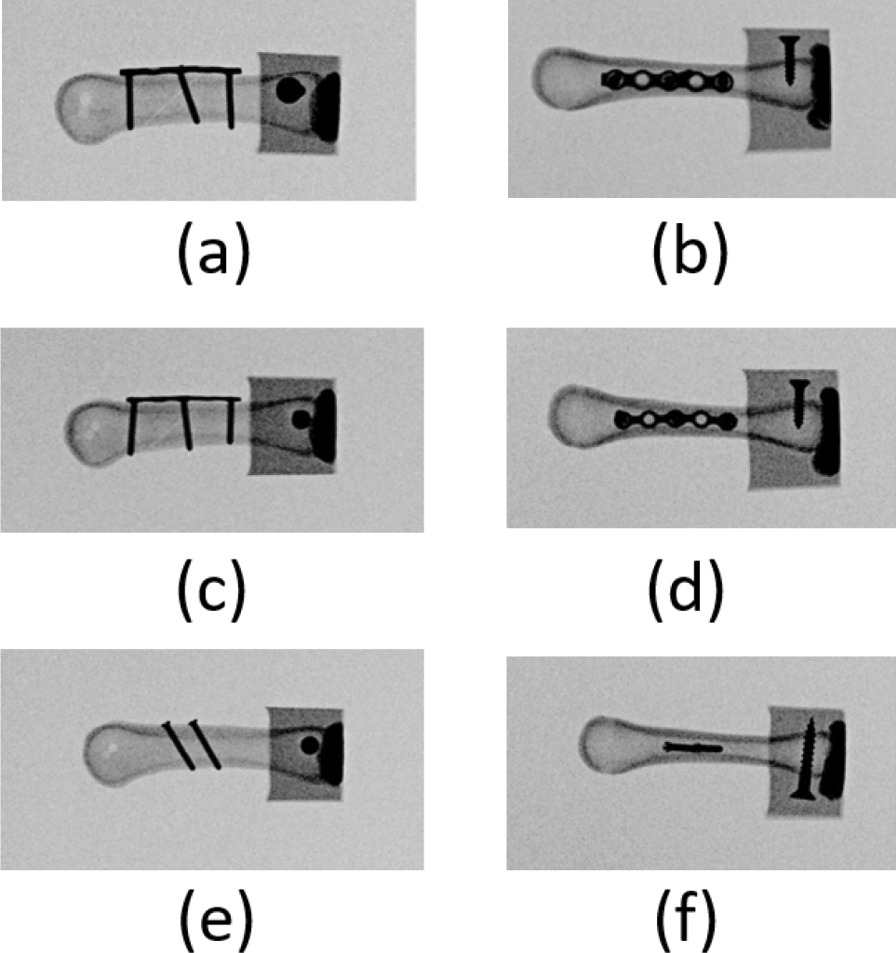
Radiographs of the three fixation approaches: regular plate fixation in a anterior–posterior view and b lateral view; locked plate fixation in c anterior–posterior view and d lateral view; and double screw fixation (e) anterior–posterior view and f lateral view
